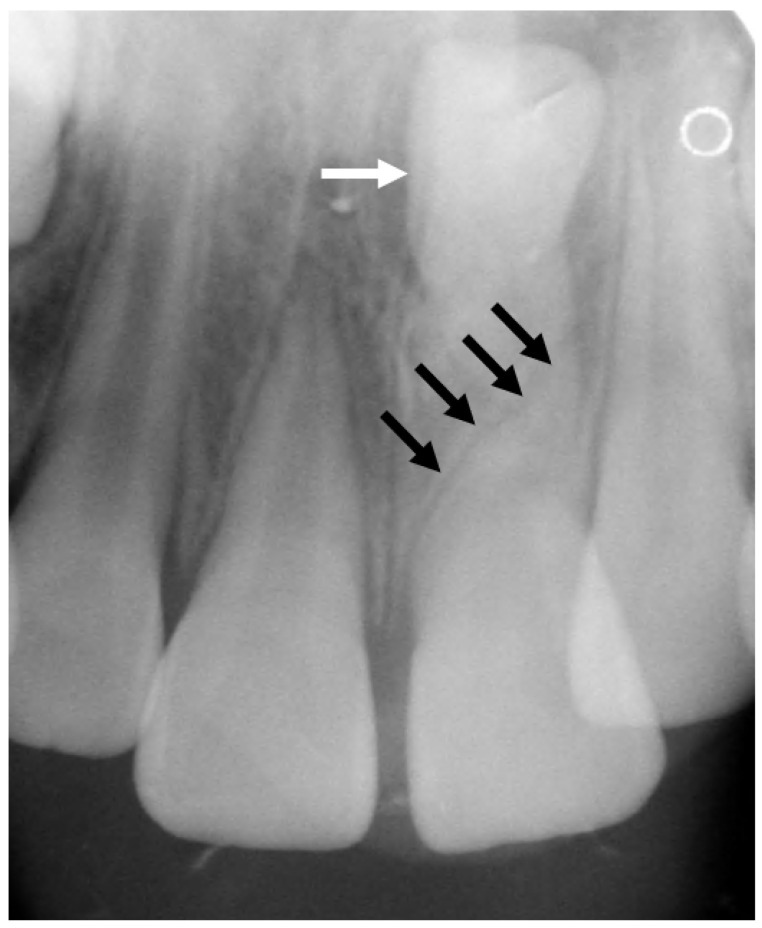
Dedicated dental radiograph demonstrating the presence of an unerupted supernumerary mesiodens (white arrow), which exerted mass effect on the left maxillary central incisor, resulting in a deformation deformity known as a dilacerated root (black arrows).
